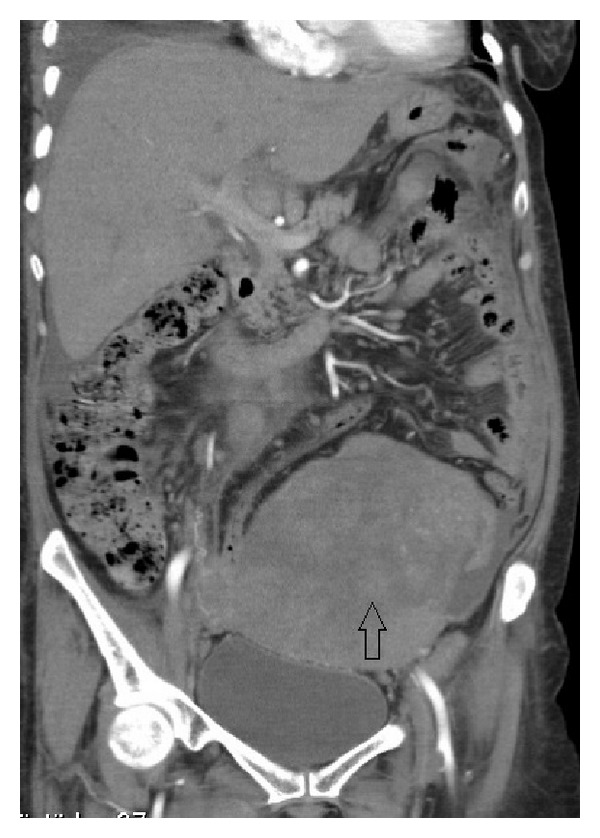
CT scan revealed a large mass of the left ovary with pelvic tissue density.
